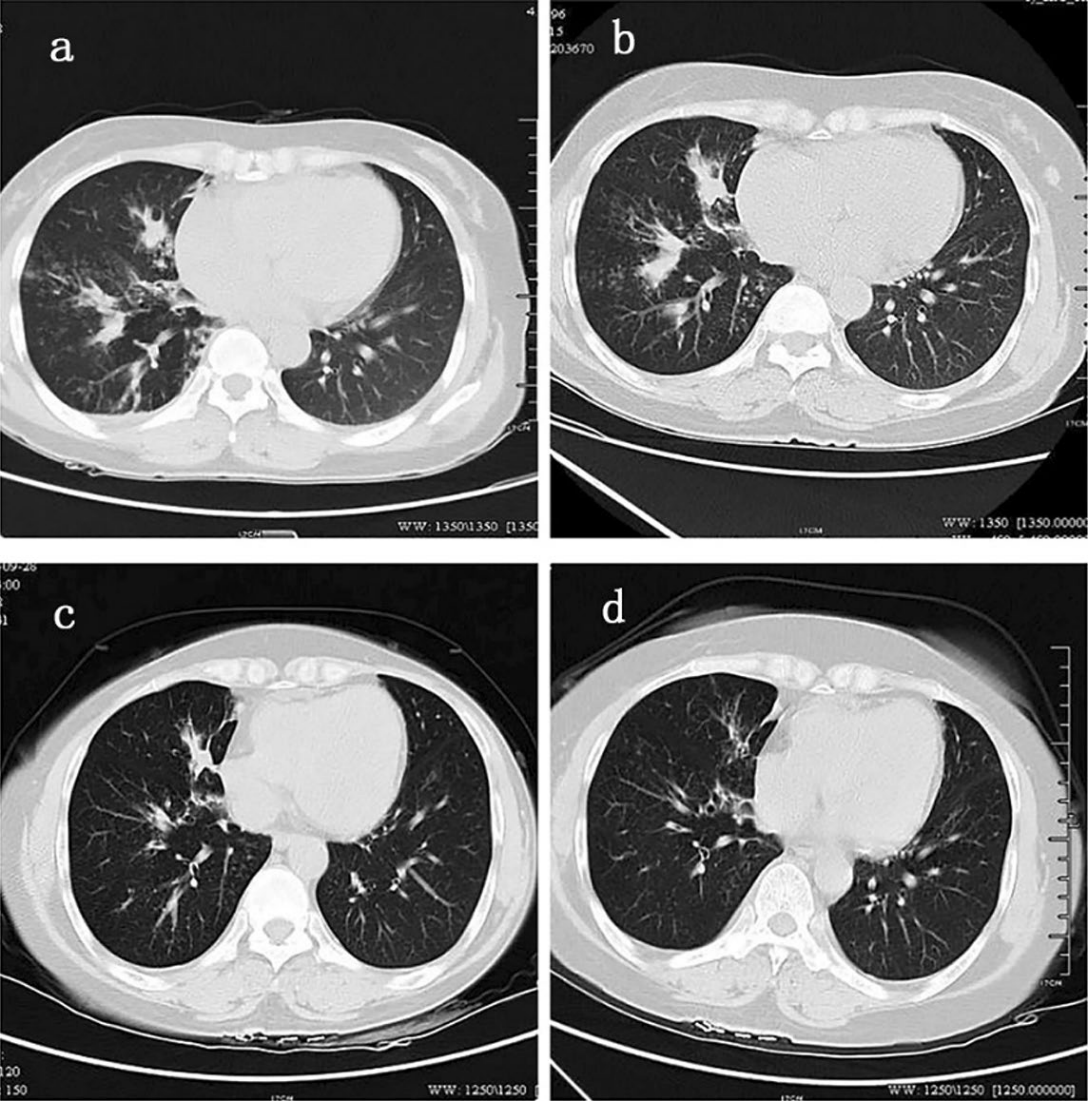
Patient underwent follow-up CT. (a) When this patient first came to our hospital, CT appeared as exudation with consolidation. (b–d) After treatment with glucocorticoids, the pulmonary consolidation significantly reduced.
